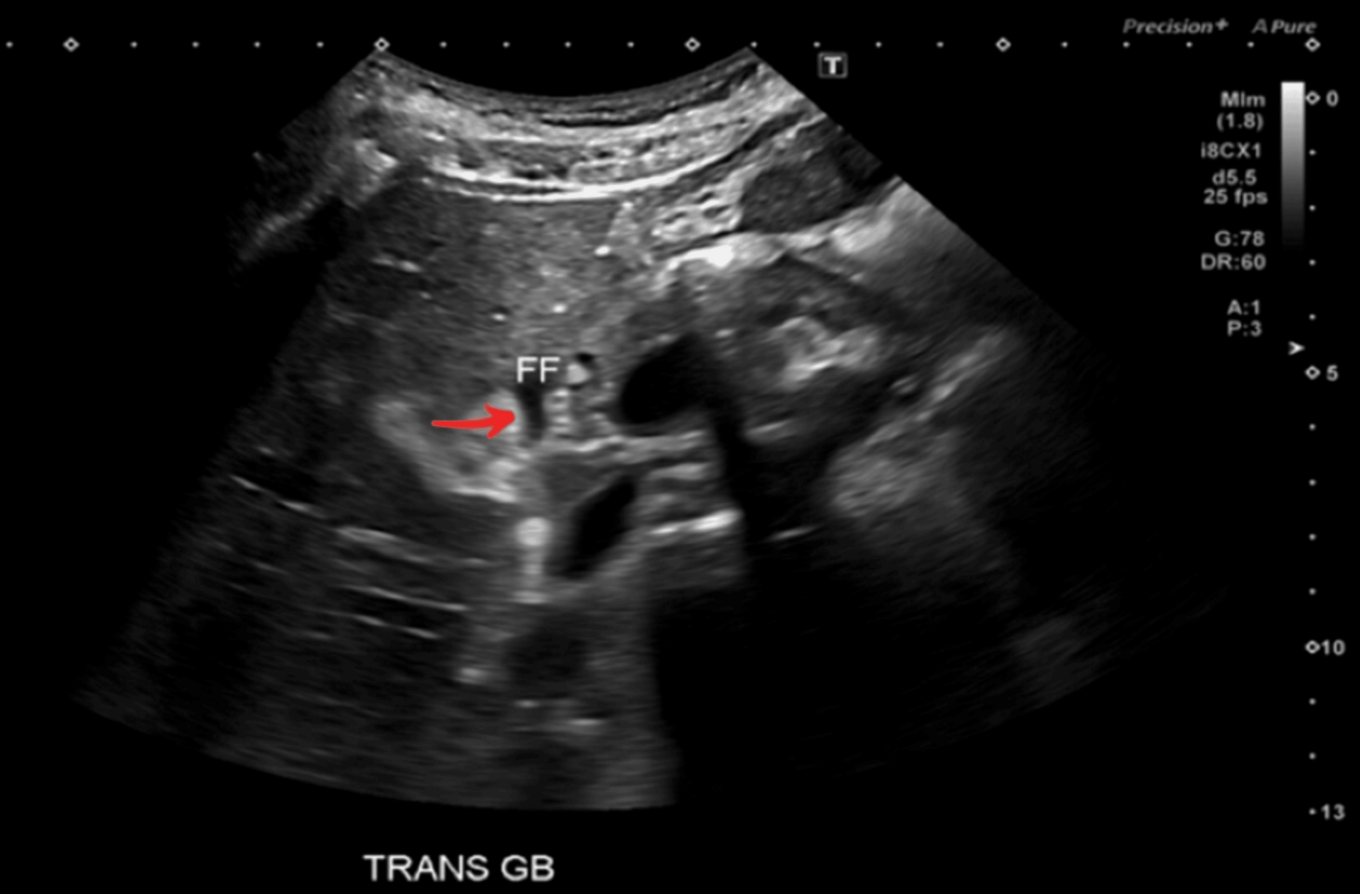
Abdominal ultrasound depicting free fluid around the gallbladder Red arrow pointing to free fluid around the gallbladder (anechoic appearance).
